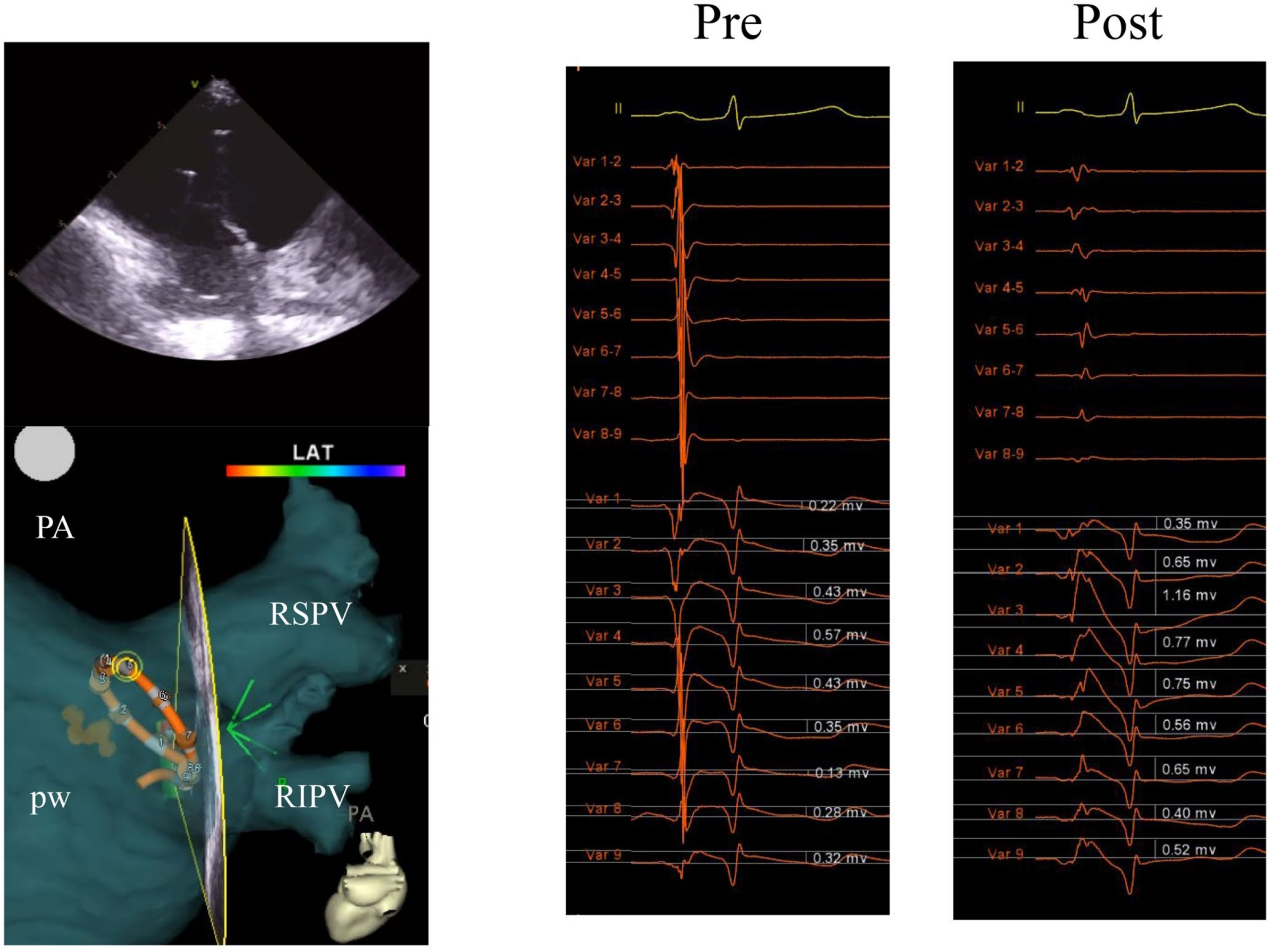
Pulsed field ablation (PFA) applied to the anterior aspect of the right superior pulmonary vein. Electrodes 4 and 5, confirmed to be in contact with myocardial tissue by intracardiac echocardiography, are highlighted as small circles on the 3D map. These electrodes demonstrated higher post‐P‐wave positive deflections on unipolar recordings compared to adjacent electrodes. Although bipolar signals disappeared after ablation, the post‐P‐wave positive deflections persisted in the unipolar electrograms.
